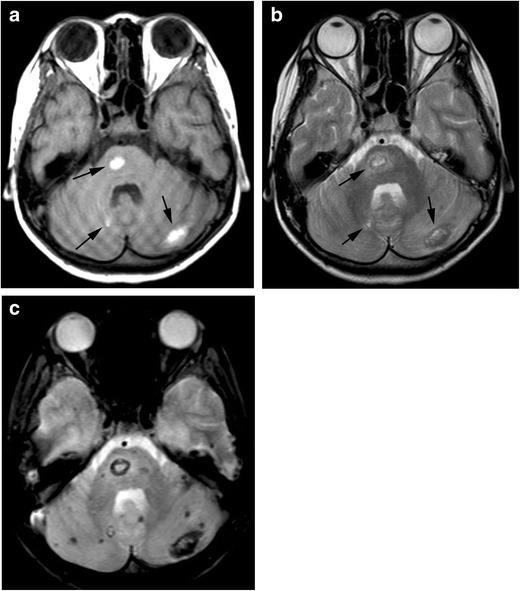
Hyperviscosity syndrome. A 10-year-old girl with acute lymphocytic leukaemia, whose white blood cell count reached 400,000, presented with headache and vomiting. a A T1-weighted image shows hyperintensity in the pons and bilateral cerebellar hemispheres (*arrows*). b These lesions show hyperintensity with a hypointensity rim on T2-weighted image (*arrows*), indicating haemorrhage in the subacute phase. c T2*-weighted image demonstrates a number of microhaemorrhages which are not seen on T1- and T2-weighted images
